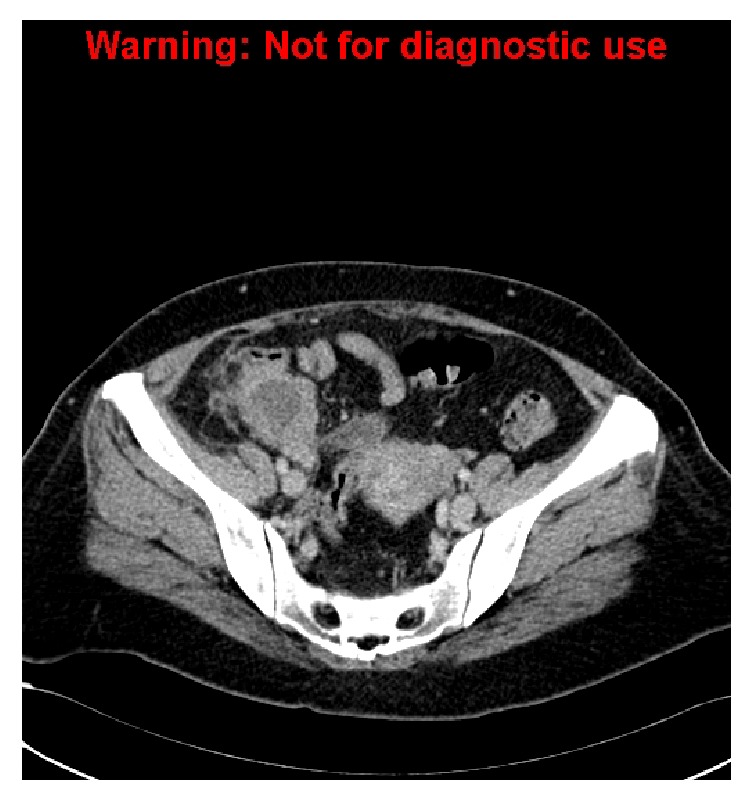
Repeat CT scan demonstrates a largely stable periappendiceal collection.
